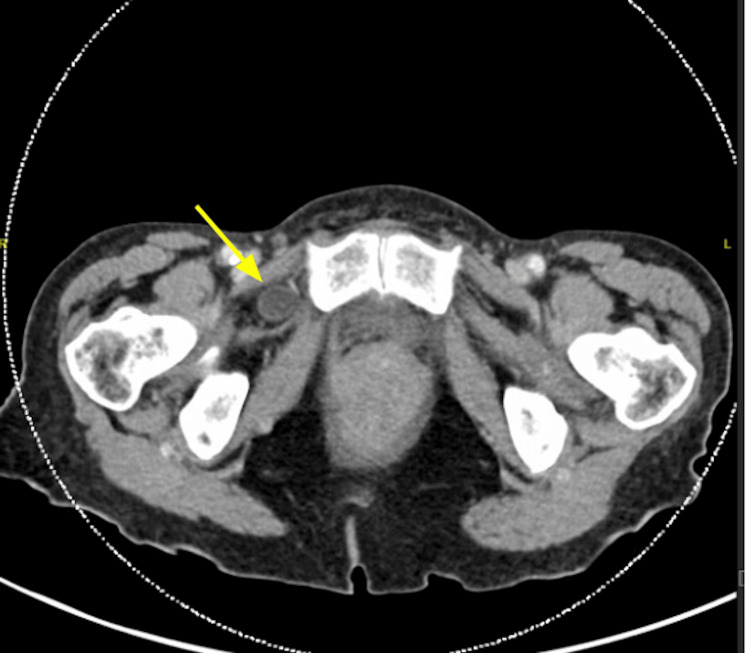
Axial CT demonstrating a right-sided obturator hernia (yellow arrow)
